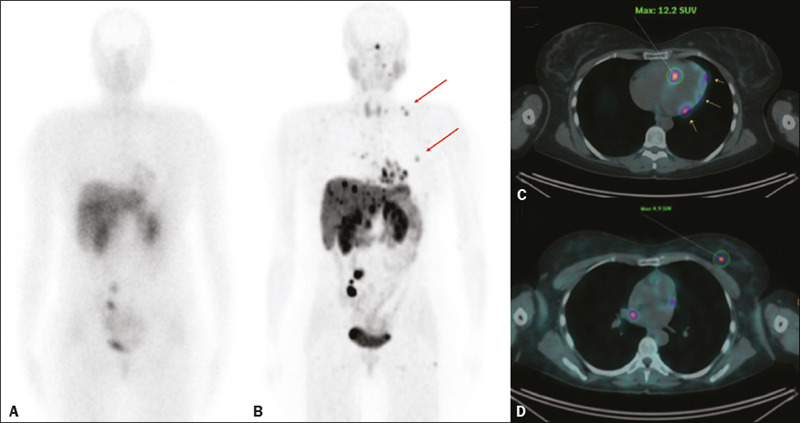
^111^In-octreotide SRS and ^68^Ga-DOTATATE PET/CT
